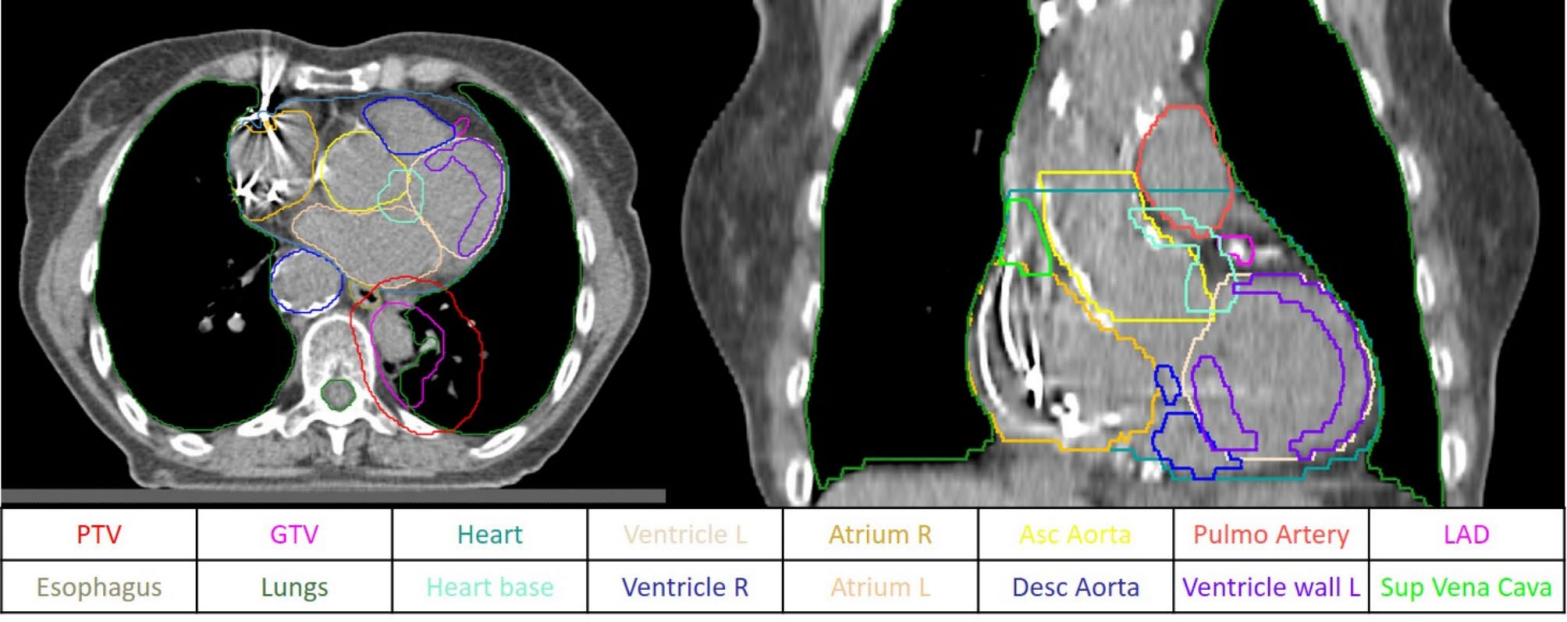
Contouring of organs at risk and cardiac substructures in axial images of planning-CT
